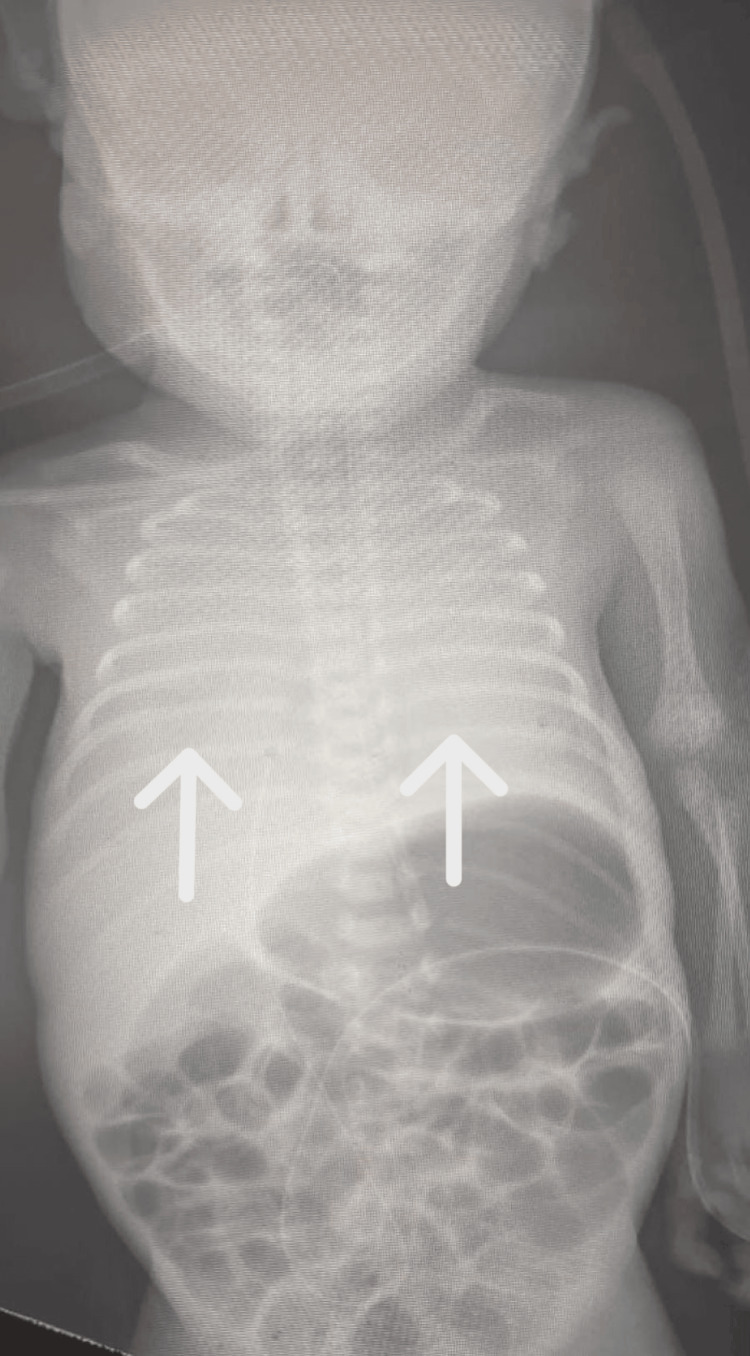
X-ray showing bilateral white lung with an endotracheal tube in the site
